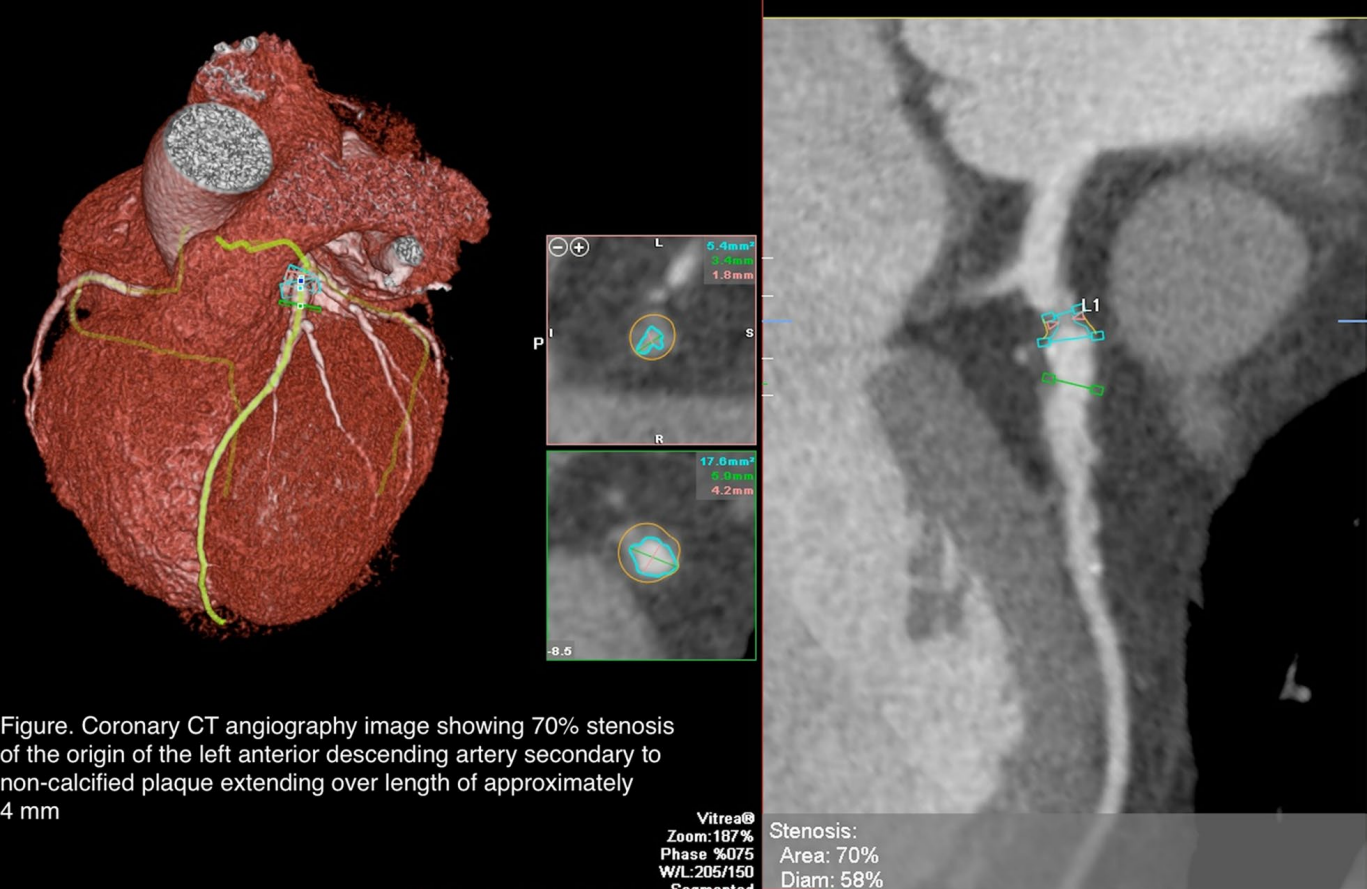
Coronary computed tomography angiography image showing 70% stenosis of the origin of the left anterior descending artery to secondary to non-calcified plaque extending over a length of approximately 4 mm
